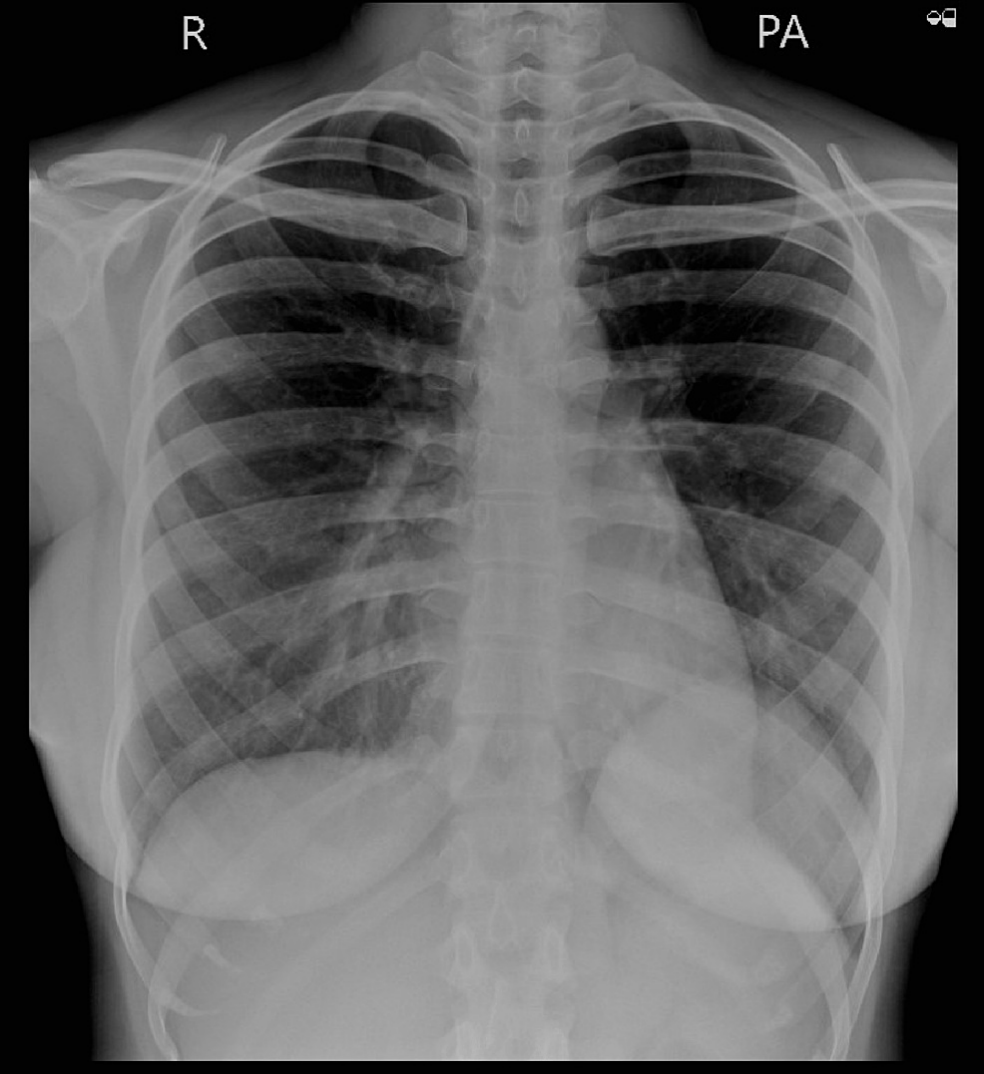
Chest radiograph PA view taken at the six-month follow-up. PA, posteroanterior
